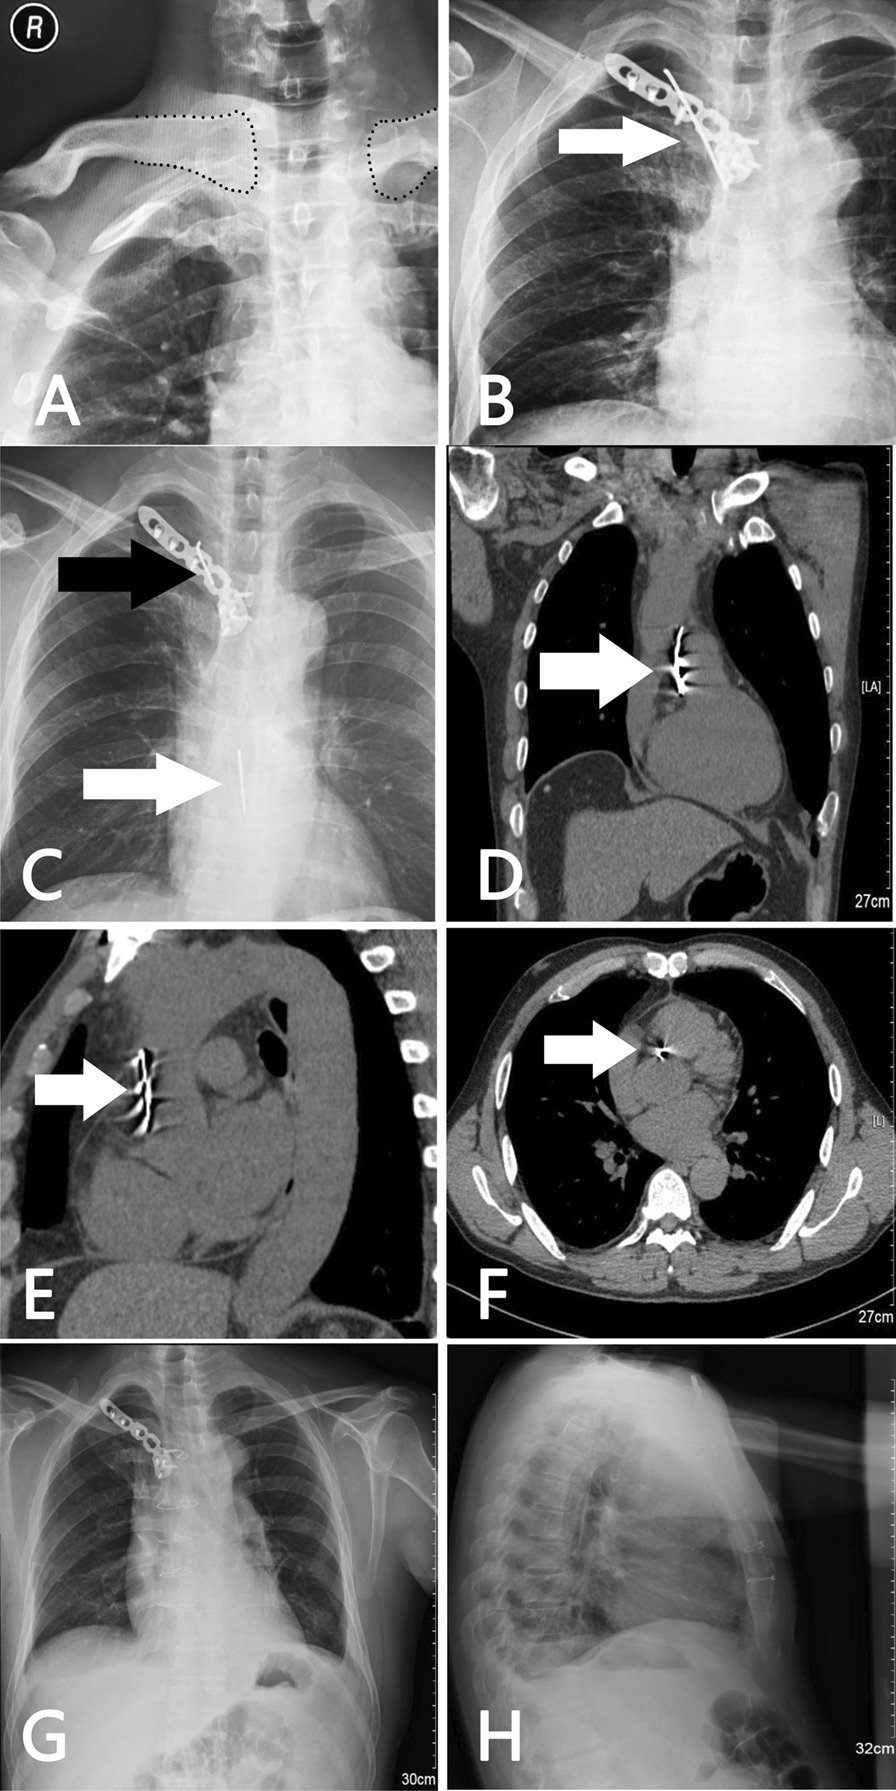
A Pre-operative radiograph of the chest showed a dislocation of the right sternoclavicular joint (dotted line). B Post-operative radiograph showed reduction of the right sternoclavicular joint with intact K-wire and locking plate. C X-ray showing a broken K-wire retained around the sternoclavicular joint (black arrow) and a metallic wire in the cardiac shadow area (white arrow) 5 months after the first operation. Anteroposterior view (D) and lateral view (E) thoracic computed tomography scan showing a linear metallic wire in the pericardium (white arrow). F Coronal view thoracic computed tomography scan showing a migrated foreign body between the aorta and right ventricle (white arrow). Postoperative posteroanterior (G) and lateral (H) chest radiographs showing the metallic wire was removed
